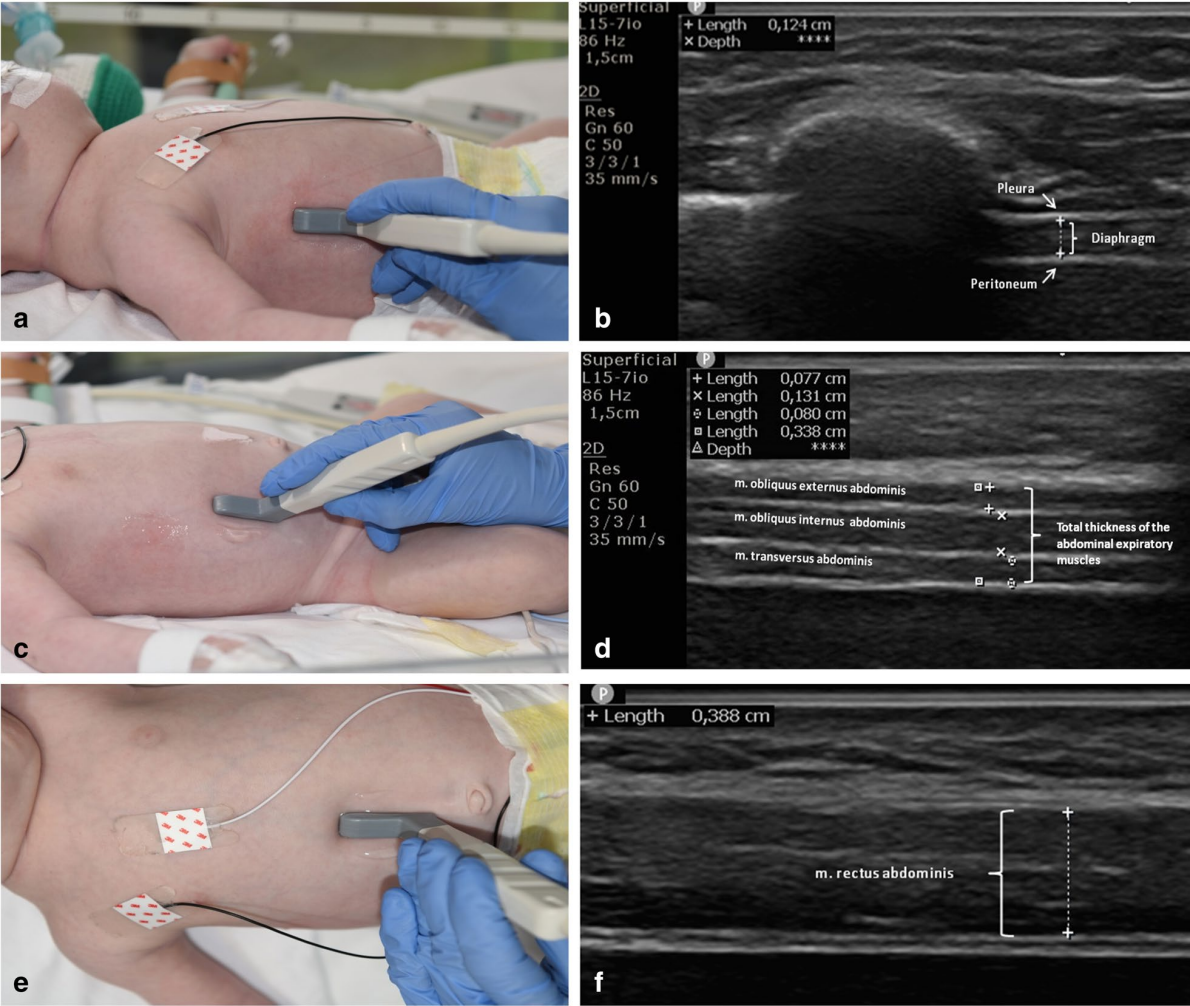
Ultrasound position and measurements of diaphragmatic thickness in the zone of apposition (a, b) of the 3 abdominal muscles (m. obliquus externus abdominis, m. obliquus internus abdominis, m. transversus abdominis), separate and together (c, d); and of the m. rectus abdominis (e, f) with a 7–15 MHz linear array transducer. Pictures were taken with the informed consent of the legal guardians
